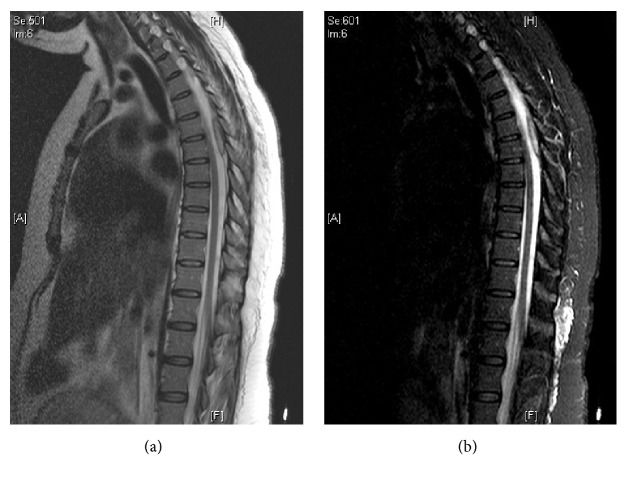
(a, b) MRI images at 14 hours postpartum showing a spinal epidural hematoma measuring 3.5 cm x 0.4 cm. There is also spinal stenosis at T5-T6 due to extrinsic mass effect of the epidural hemorrhage.
